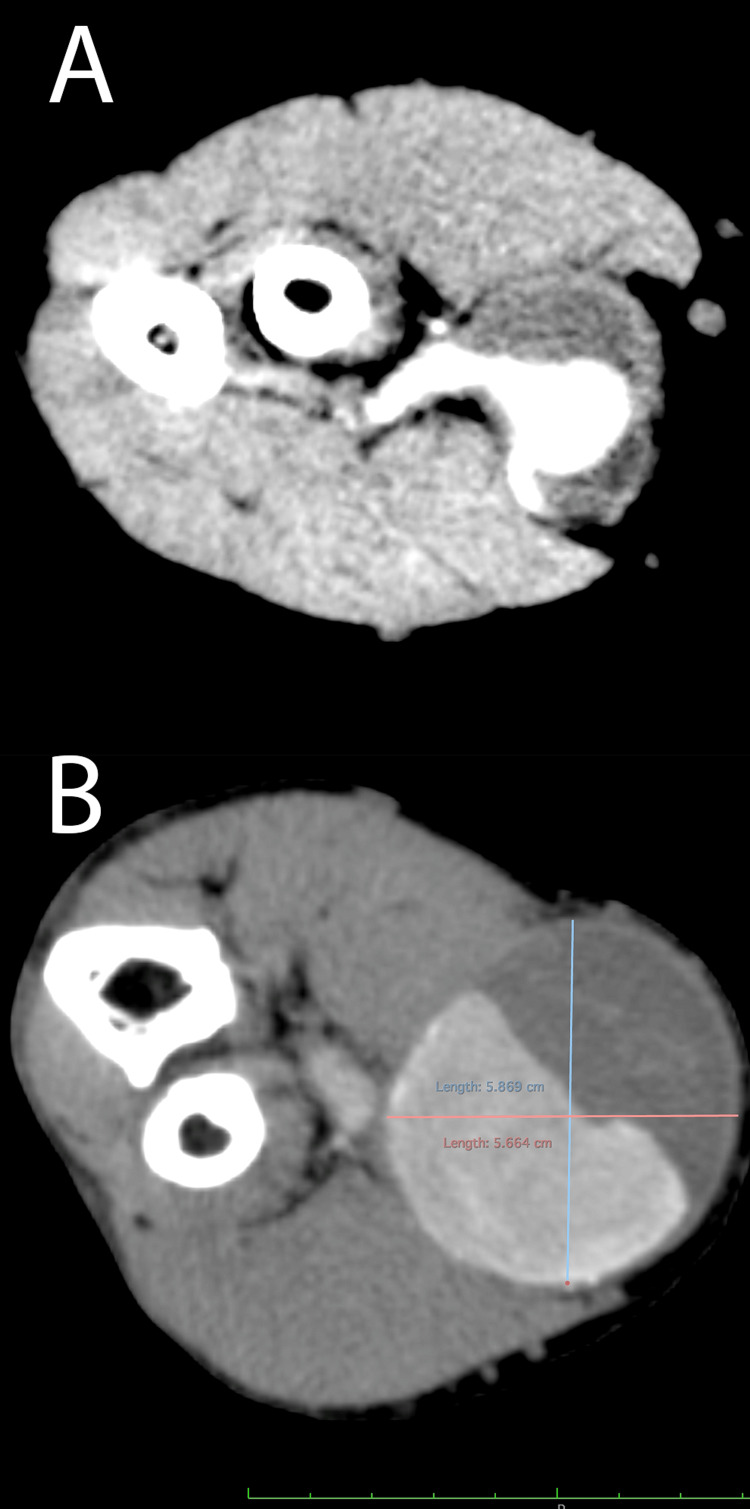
Preoperative computed tomography angiography, depicting (A) the saccular shape of the brachial artery aneurysm and (B) the maximum sac dimensions of the brachial artery aneurysm
